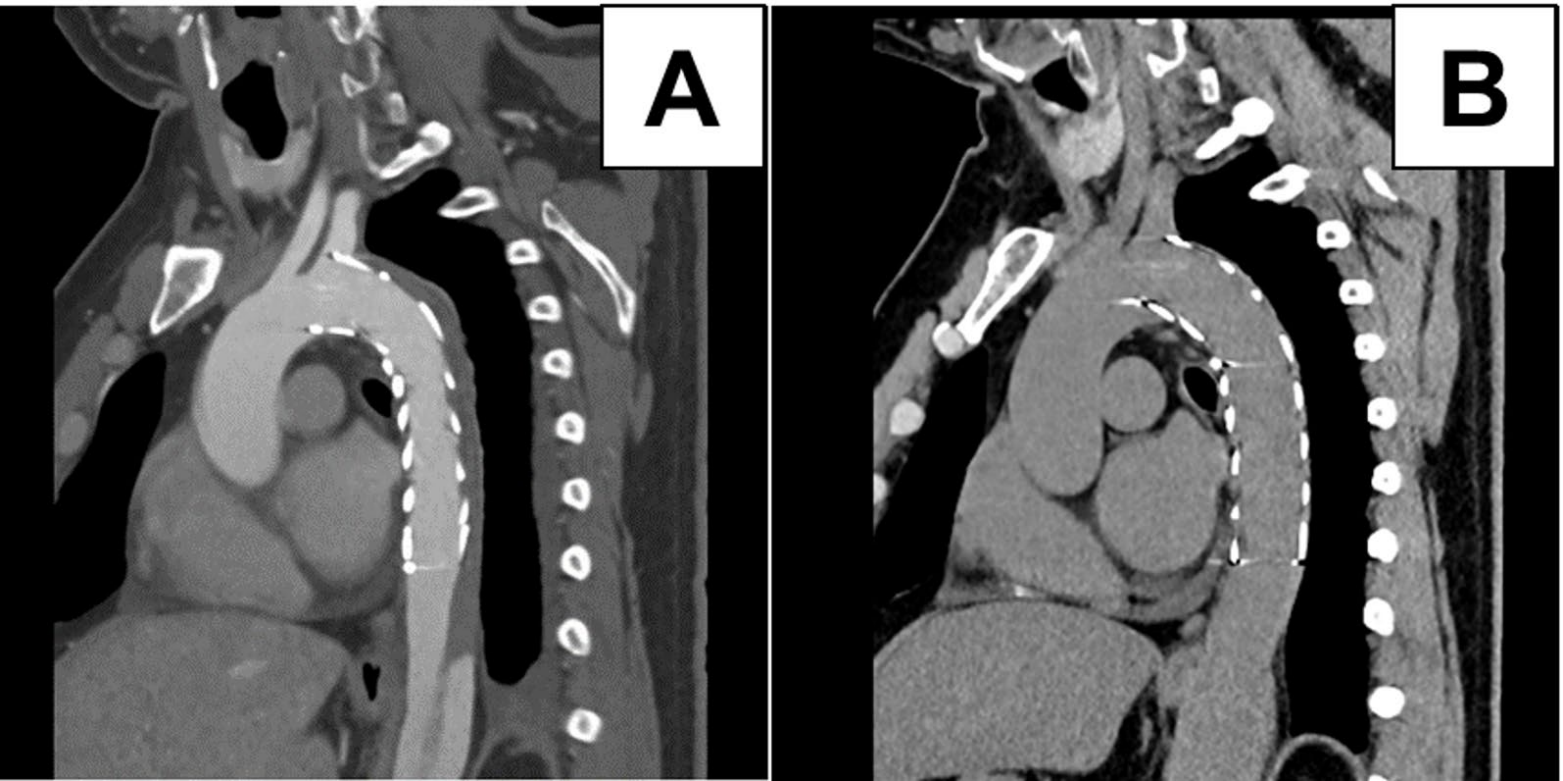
Postoperative contrast-enhanced computed tomography (CT) images after TEVAR. (A) CT obtained 4 days postoperatively shows complete exclusion of the primary entry tear and thrombosis of the false lumen in the thoracic aorta. (B) CT at 1 year follow-up demonstrates sustained false lumen thrombosis and favorable aortic remodeling with preserved LSA perfusion
